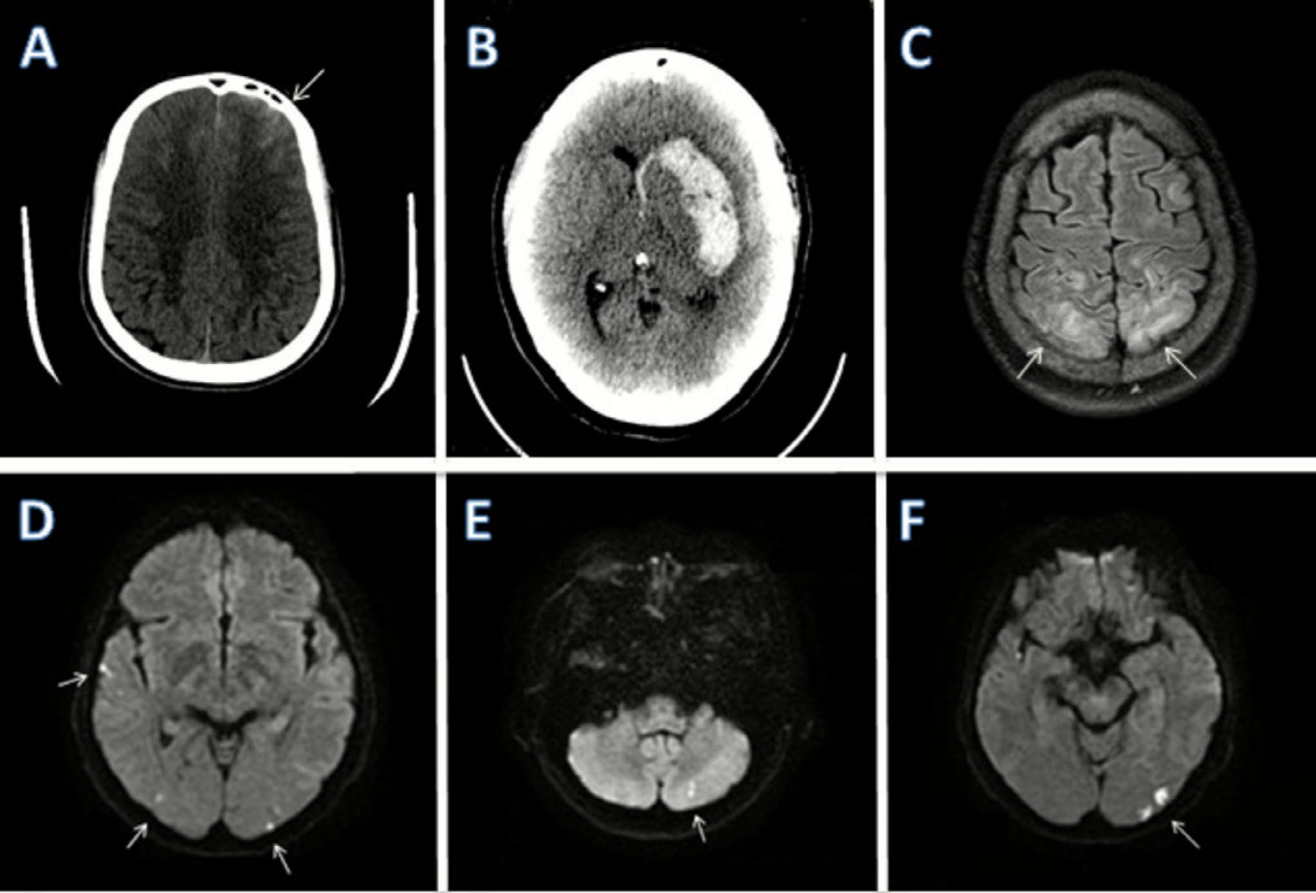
Typical parenchymal findings in patients with RCVS. Brain CT showing a left frontal convexity subarachnoid hemorrhage (A) and a left basal nuclei hematoma with ventricular extension (white arrows) (B). Brain MRI on the FLAIR sequence showing bilateral hyperintensity in the posterior territories, suggestive of vasogenic edema (C). The DWI sequence shows multiple diffusion restriction foci in cortical, subcortical, and watershed areas (white arrows), observed in the right temporal and bilateral occipital lobes (D), the left cerebellar hemisphere (E), and the left occipital lobe (F). CT, computed tomography; DWI, diffusion‐weighted imaging; FLAIR, fluid‐attenuated inversion recovery; MRI, magnetic resonance imaging. [Color figure can be viewed at wileyonlinelibrary.com]
